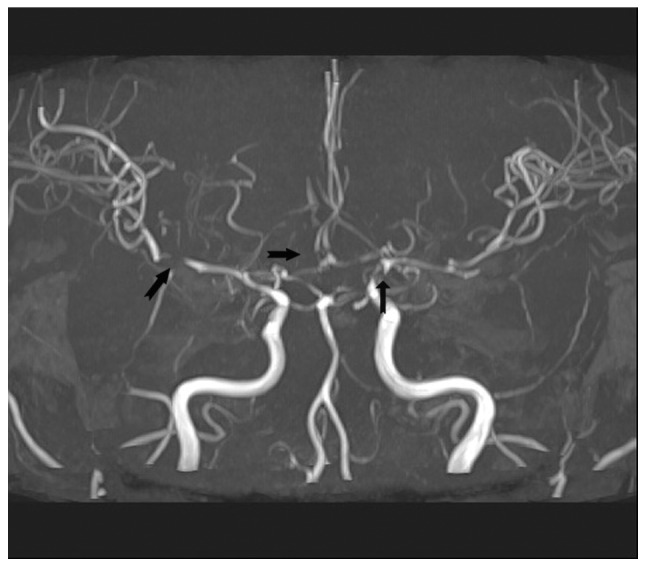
Brain magnetic resonance image demonstrates the blood vessel damage (arrows) due to the severe hypertension.
